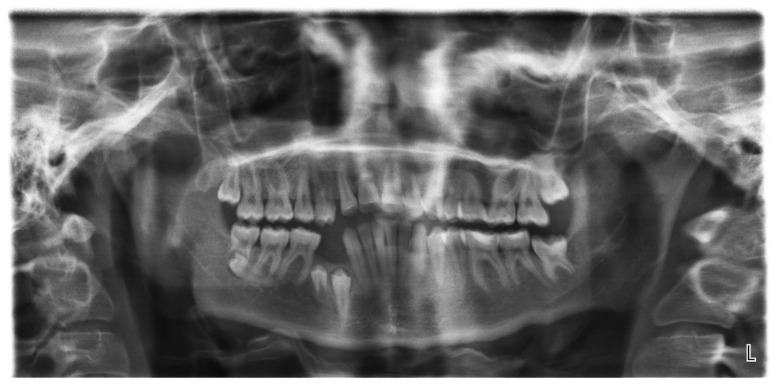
Panoramic radiograph, taken two years later, when patient was 15 years old, showing the further distal migration of the tooth 45 above the mandibular canal (its crown can be seen to overlap the roots of the tooth 48), as well as the still unerupted impacted tooth 44 and the third additional tooth.
